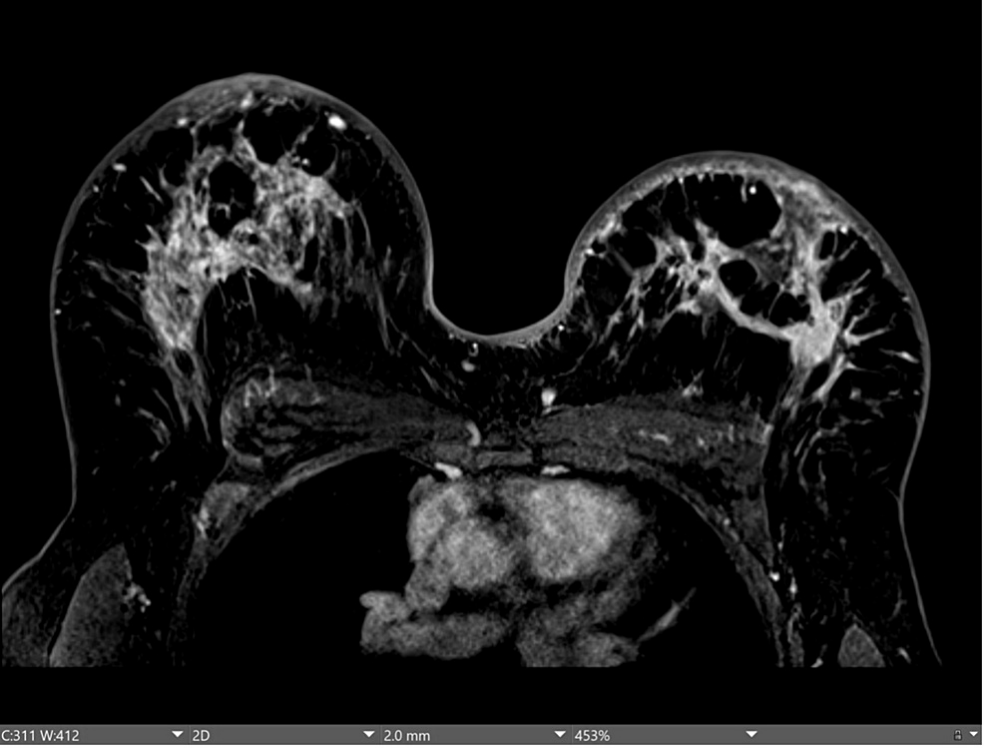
T1-weighted axial contrast-enhanced MRI demonstrating skin thickening of the right and left breasts demonstrating bilateral breast masses and abnormal enhancement. MRI: magnetic resonance imaging
